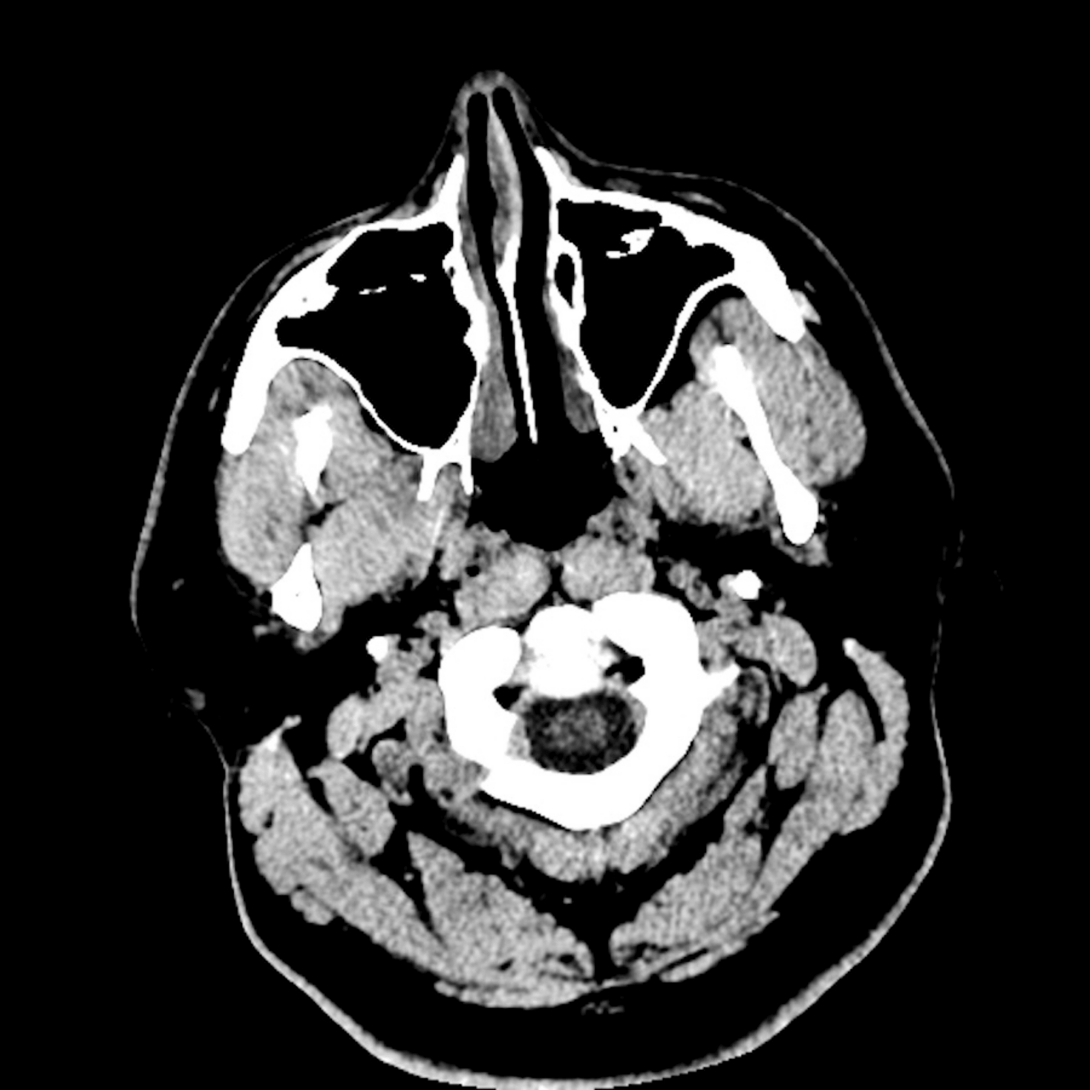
Normal non-contrast computed tomography of the brain No acute intracranial hemorrhage, midline shift, or mass effect.
